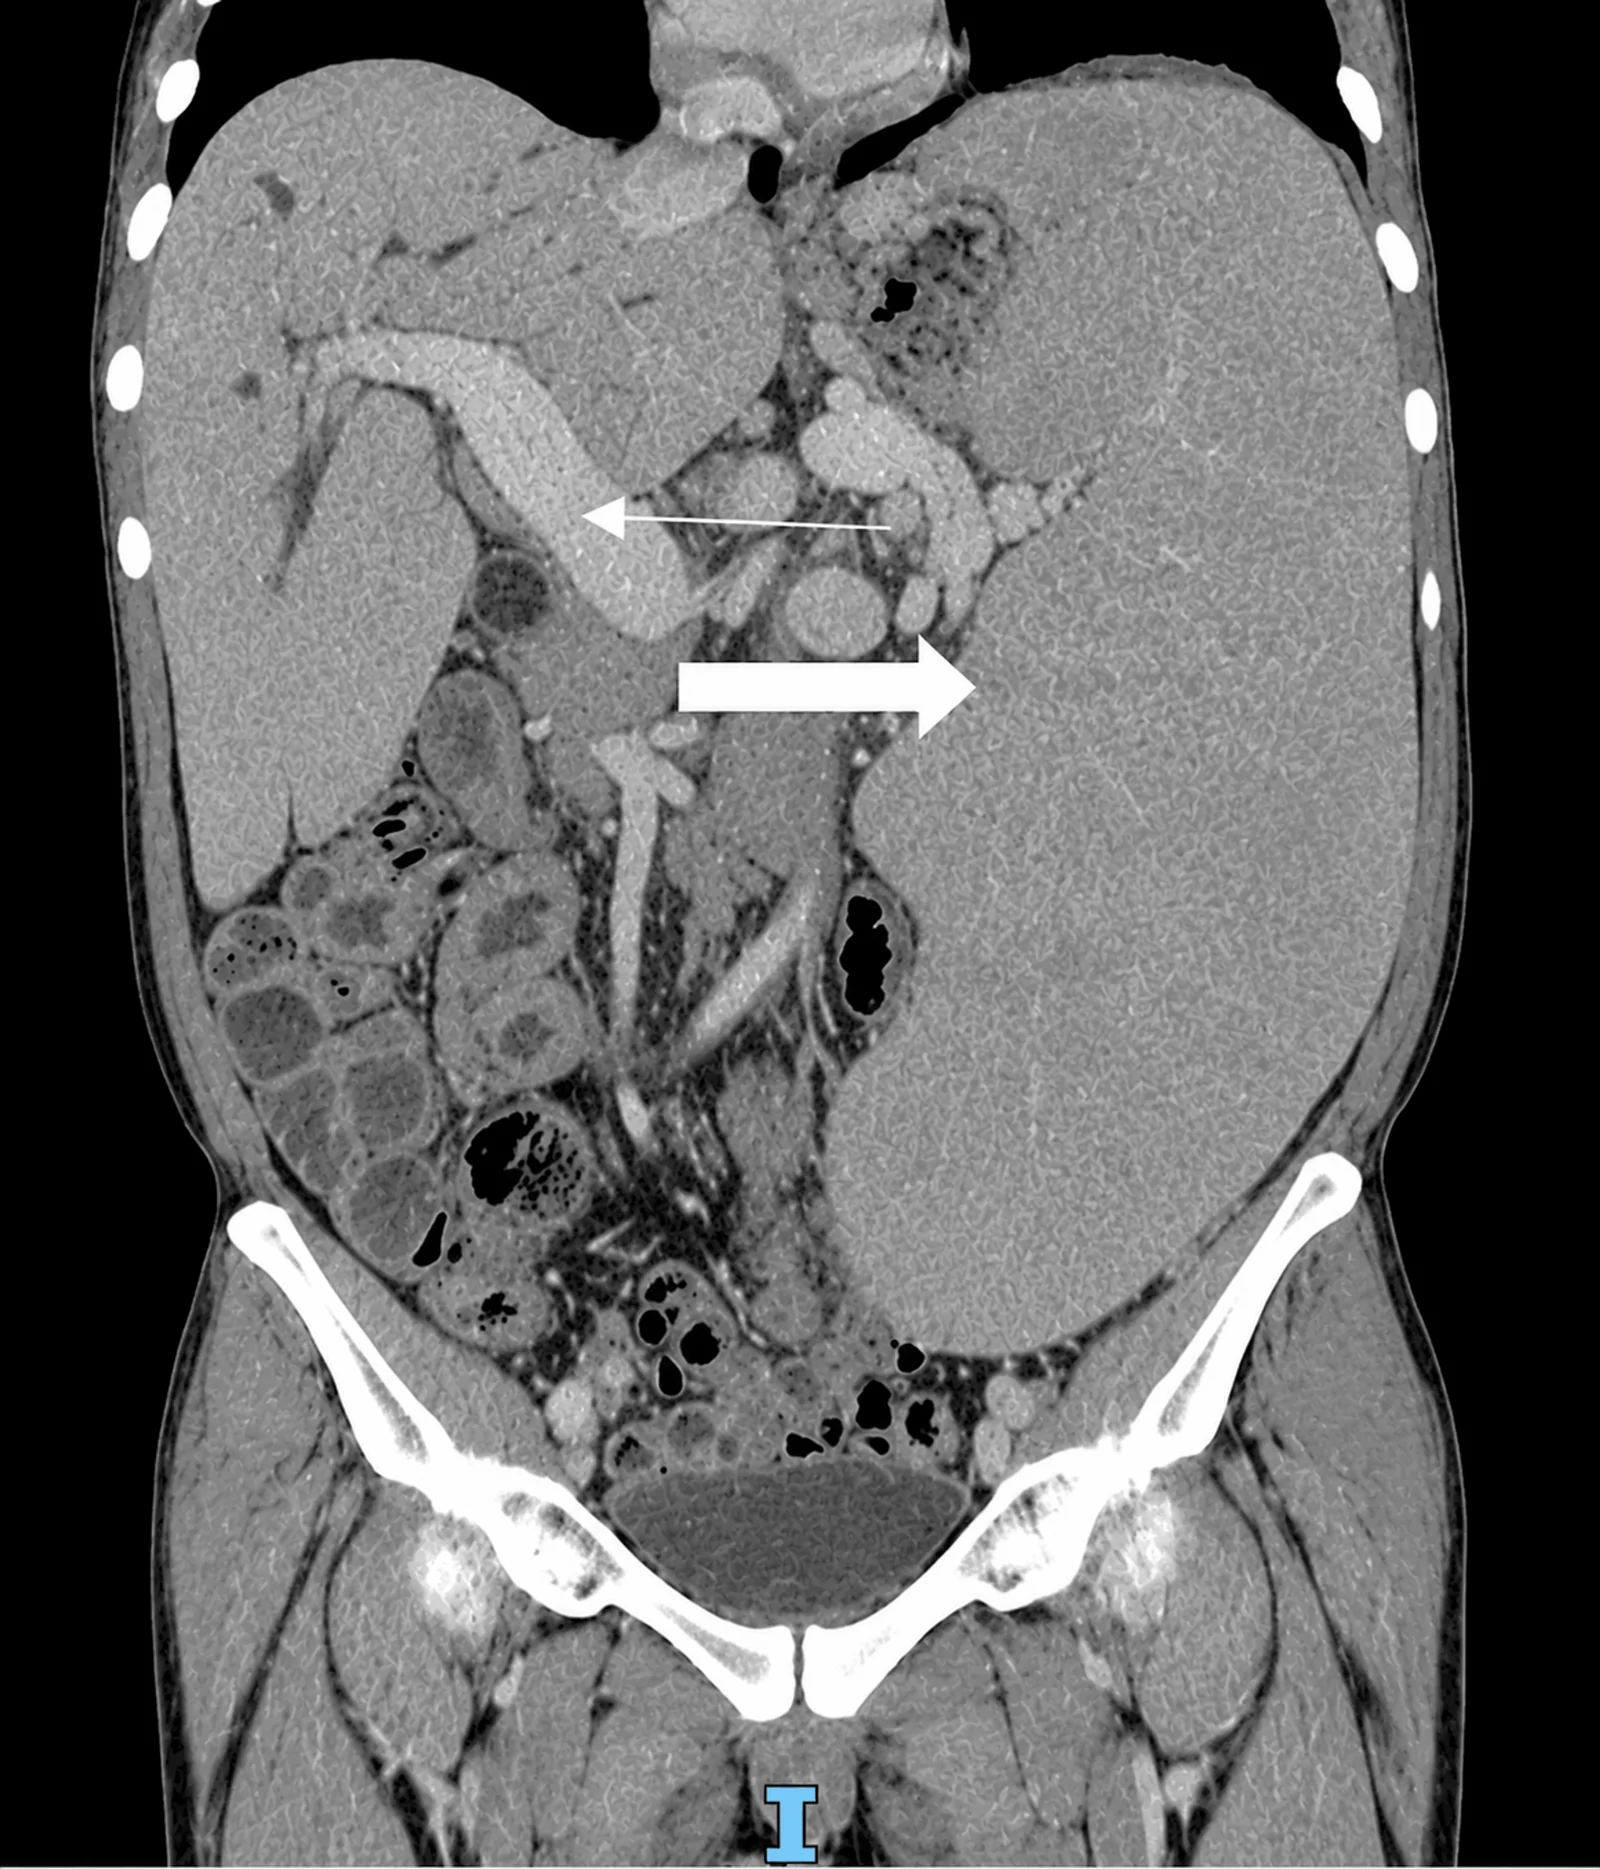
Contrast-enhanced abdominal CT Demonstrating hepatomegaly with signs of portal hypertension (thin white arrow) and massive splenomegaly extending from the left upper quadrant to the left iliac fossa, with an estimated splenic volume of 6,100 mL (thick white arrow). CT: computed tomography
